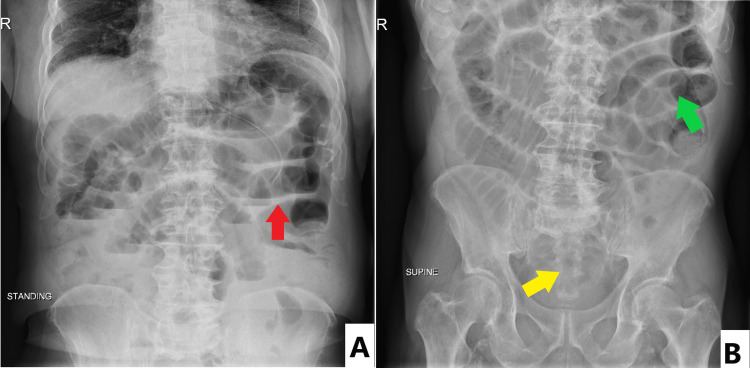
Abdominal x-ray in the standing (A) and supine (B) positions showing air-fluid levels in the standing position and distended small bowel loops in the supine position. The absence of air in the large bowels and rectum also suggest an obstruction in the small bowels. Red arrow: air-fluid levels; Green arrow: distended small bowels; Yellow arrow: absence of air in rectum
